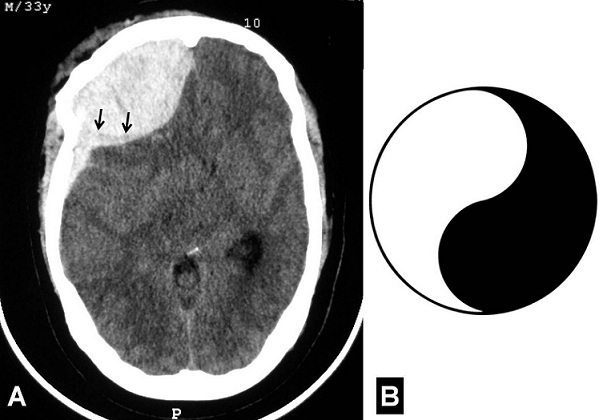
A) Axial CT-scan showing extraaxial hemorrhagic lesion on the right frontoparietal region. This lesion has two different components: the first (anterior) was epidural and the second (posterior) was subdural. Note the limit within this lesion (arrows); B) The Yin-yang symbol or Taijitu
